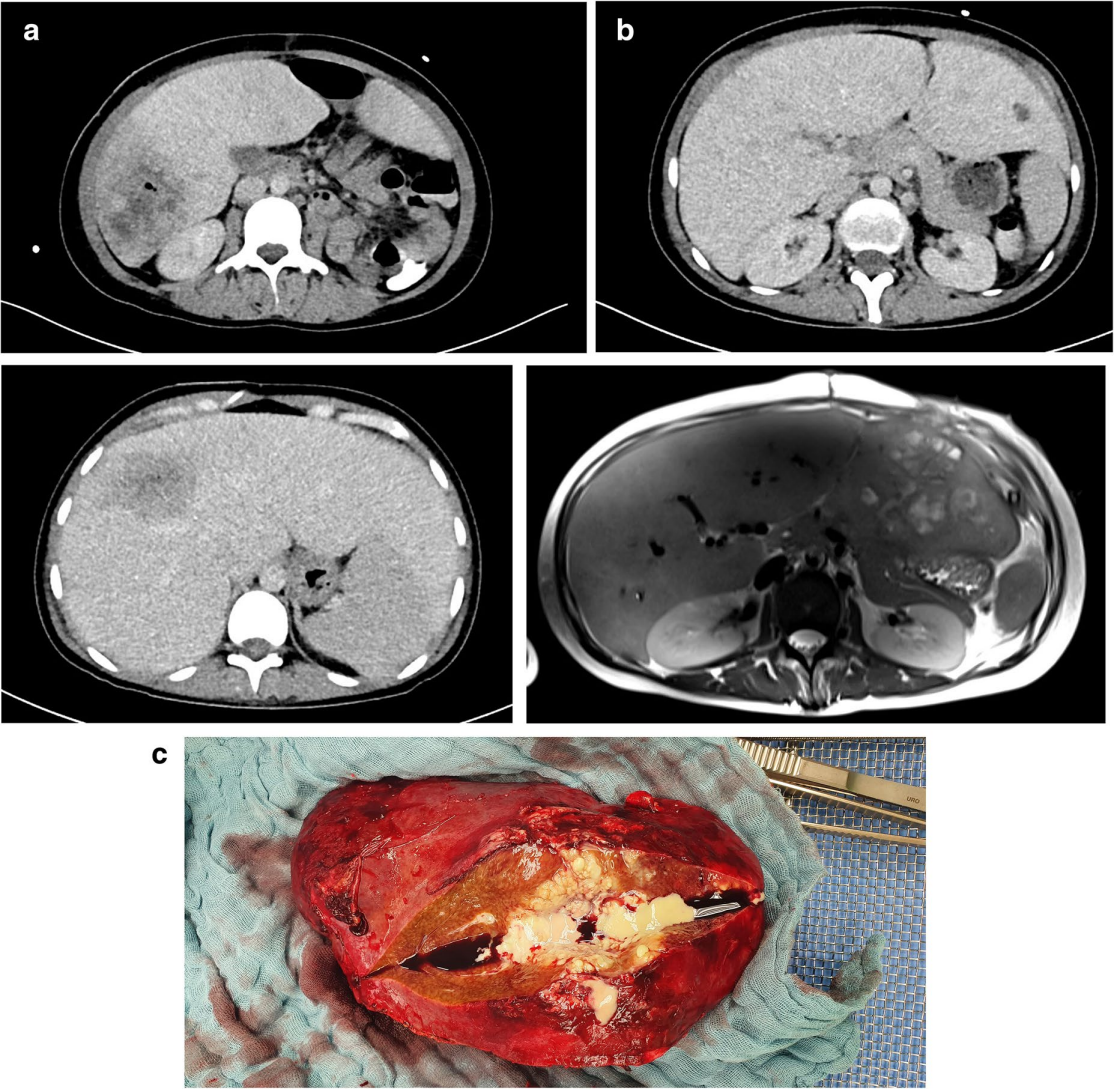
Panel a shows the initial CT of a female 29-year-old patient with multiple located PLA and inflammatory bowel disease. An interenteric fistula could be found as the infectious origin. Colon segment resection, repeated interventional drainage, and long-term antibiotic treatment were performed over 7 weeks. Lastly, PLA was narrowed to the left-lateral liver lobe (Panel b), and liver resection was performed in a final step (Panel c). The patient regenerated fully and had no PLA recurrence. This underlines the complexity of PLA treatment and the usefulness of interdisciplinary cooperation
